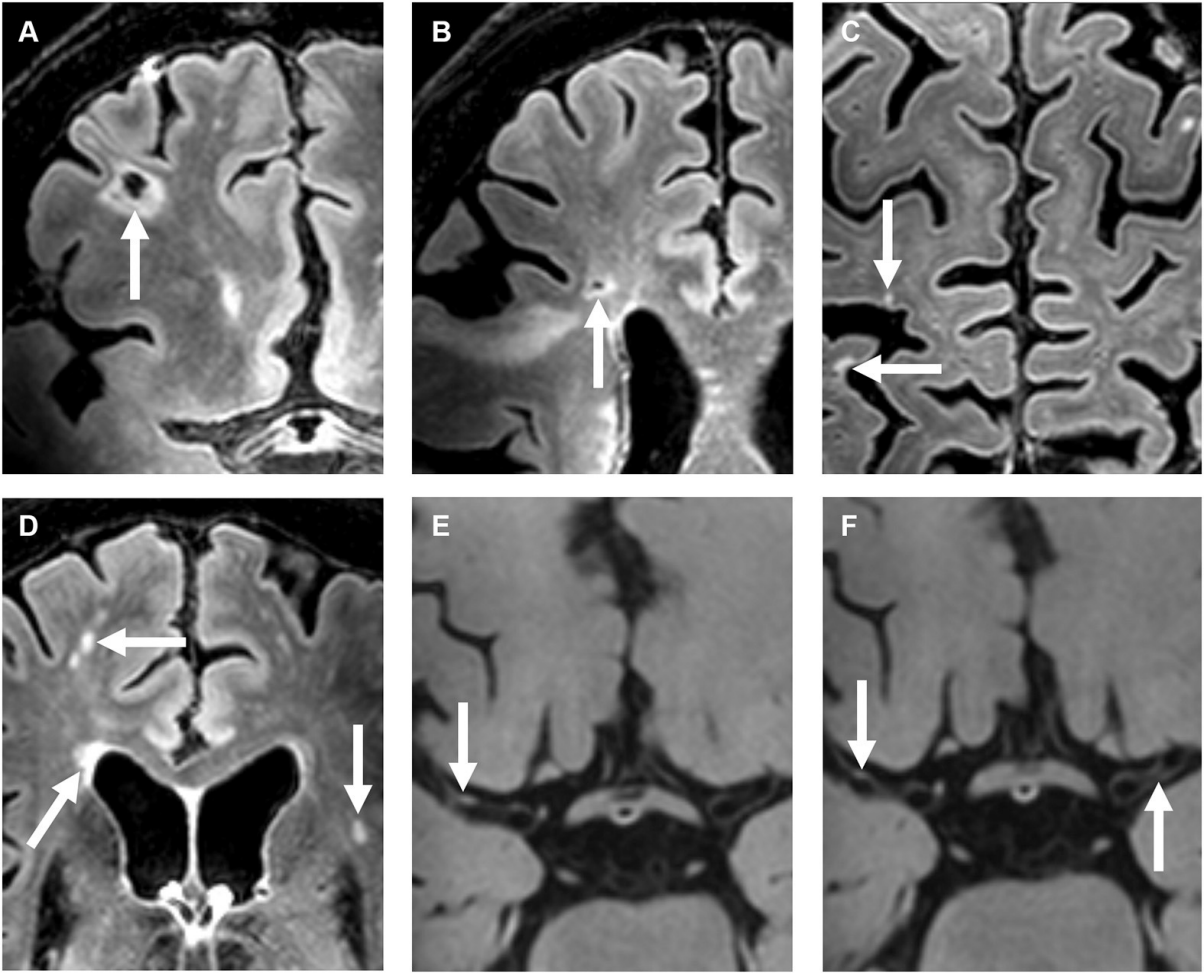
A 72-year-old female with a right-sided ischemic stroke; imaging 25 days after symptom onset. (A–D) Precontrast 7T transverse FLAIR images and (E–H) post-contrast 7T transverse T_1_-weighted MPIR-TSE vessel wall images. Lacunar infarcts are located in the right frontal [arrow in (A)] and right frontotemporal area [arrow in (B)]. Multiple cortical microinfarcts are also seen [arrows in (C)], as well as periventricular and deep white matter hyperintensities [arrows in (D)]. Note also the extensive enlarged periventricular spaces in this patient [not scored; arrows in (E,F)]. Vessel wall lesions are seen in the left and right middle cerebral arteries (M1 segments) [arrows in (G,H)].
